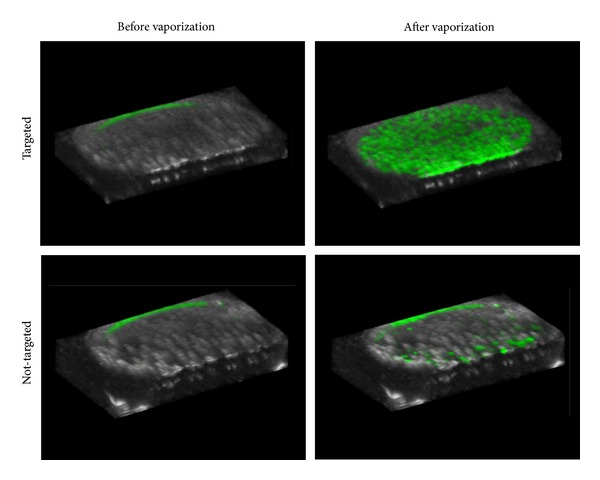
Overlays of contrast-specific CPS intensity (green scale) and traditional B-mode (grey scale) ultrasound scans: 3-D rendering of the ultrasound slices across the cell sample volume reveals the contrast enhancement provided by targeted PCAs after the activation pulse. Non-targeted samples resulted in a small amount of contrast enhancement, likely as a result of non-selective binding. The small amount of contrast present at the top of each sample is an artifact of the metal holder, not a result of the presence of echogenic bubbles. Reprinted from [99], Copyright 2013, with permission from Elsevier.
